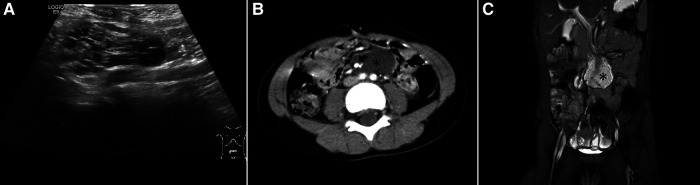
Imaging of a patient with a complicated cyst located at the root of the intestinal mesentery. (A) Sonograph demonstrating a hypoechoic cyst with multiple vehicles and internal septations; (B) enhanced CT scan showing a low-density cyst (asterisk) located at the root of the intestinal mesentery and surrounded mesenteric vessels (arrow); (C) MRI showing a cyst with significant signal intensities on T2-weighted imaging.
